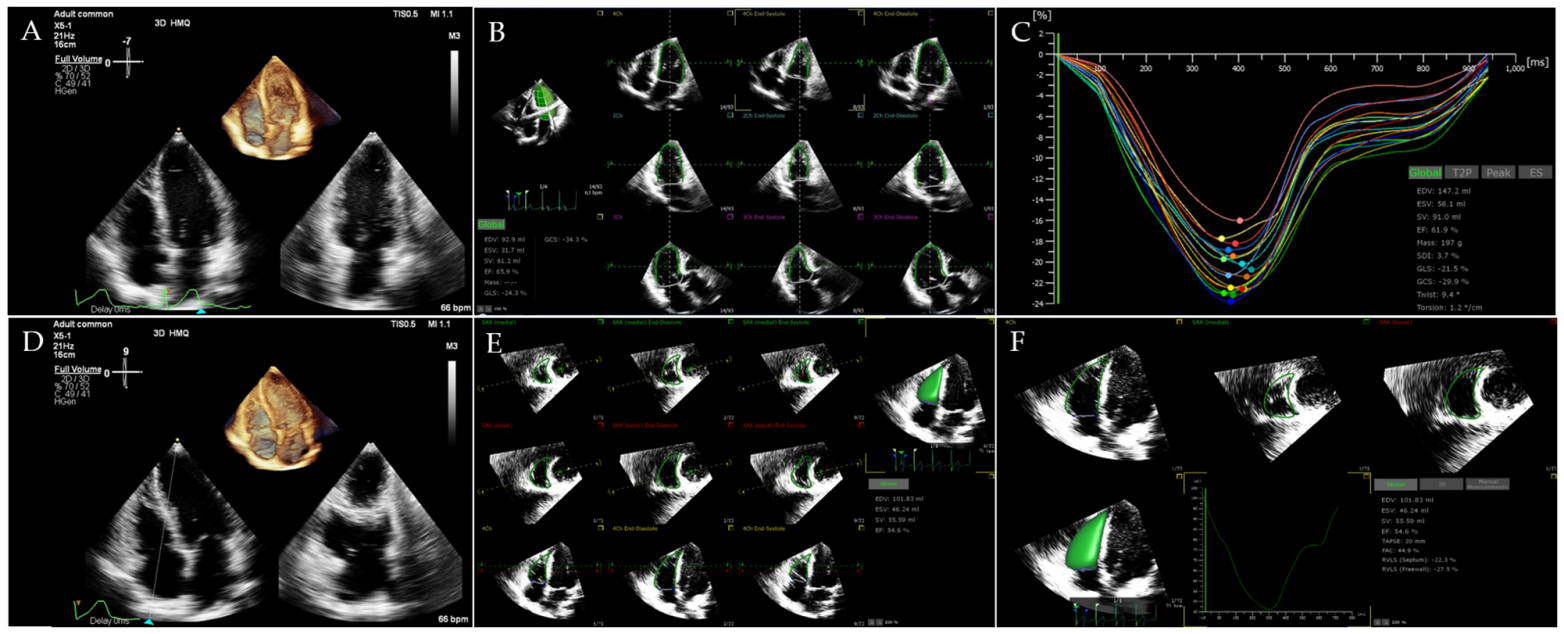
Ventricular three-dimensional image. (A) Three-dimensional image of LV-focused apical 4-chamber view. (B) LV endocardial border identification and tracking at end-systole and end-diastole. (C) Longitudinal strain of LV. (D) Three-dimensional image of RV-focused apical 4-chamber view. (E) RV endocardial border identification and tracking at end-systole and end-diastole. (F) Longitudinal strain of RV free wall and RV volume-time curve were automatically generated.
